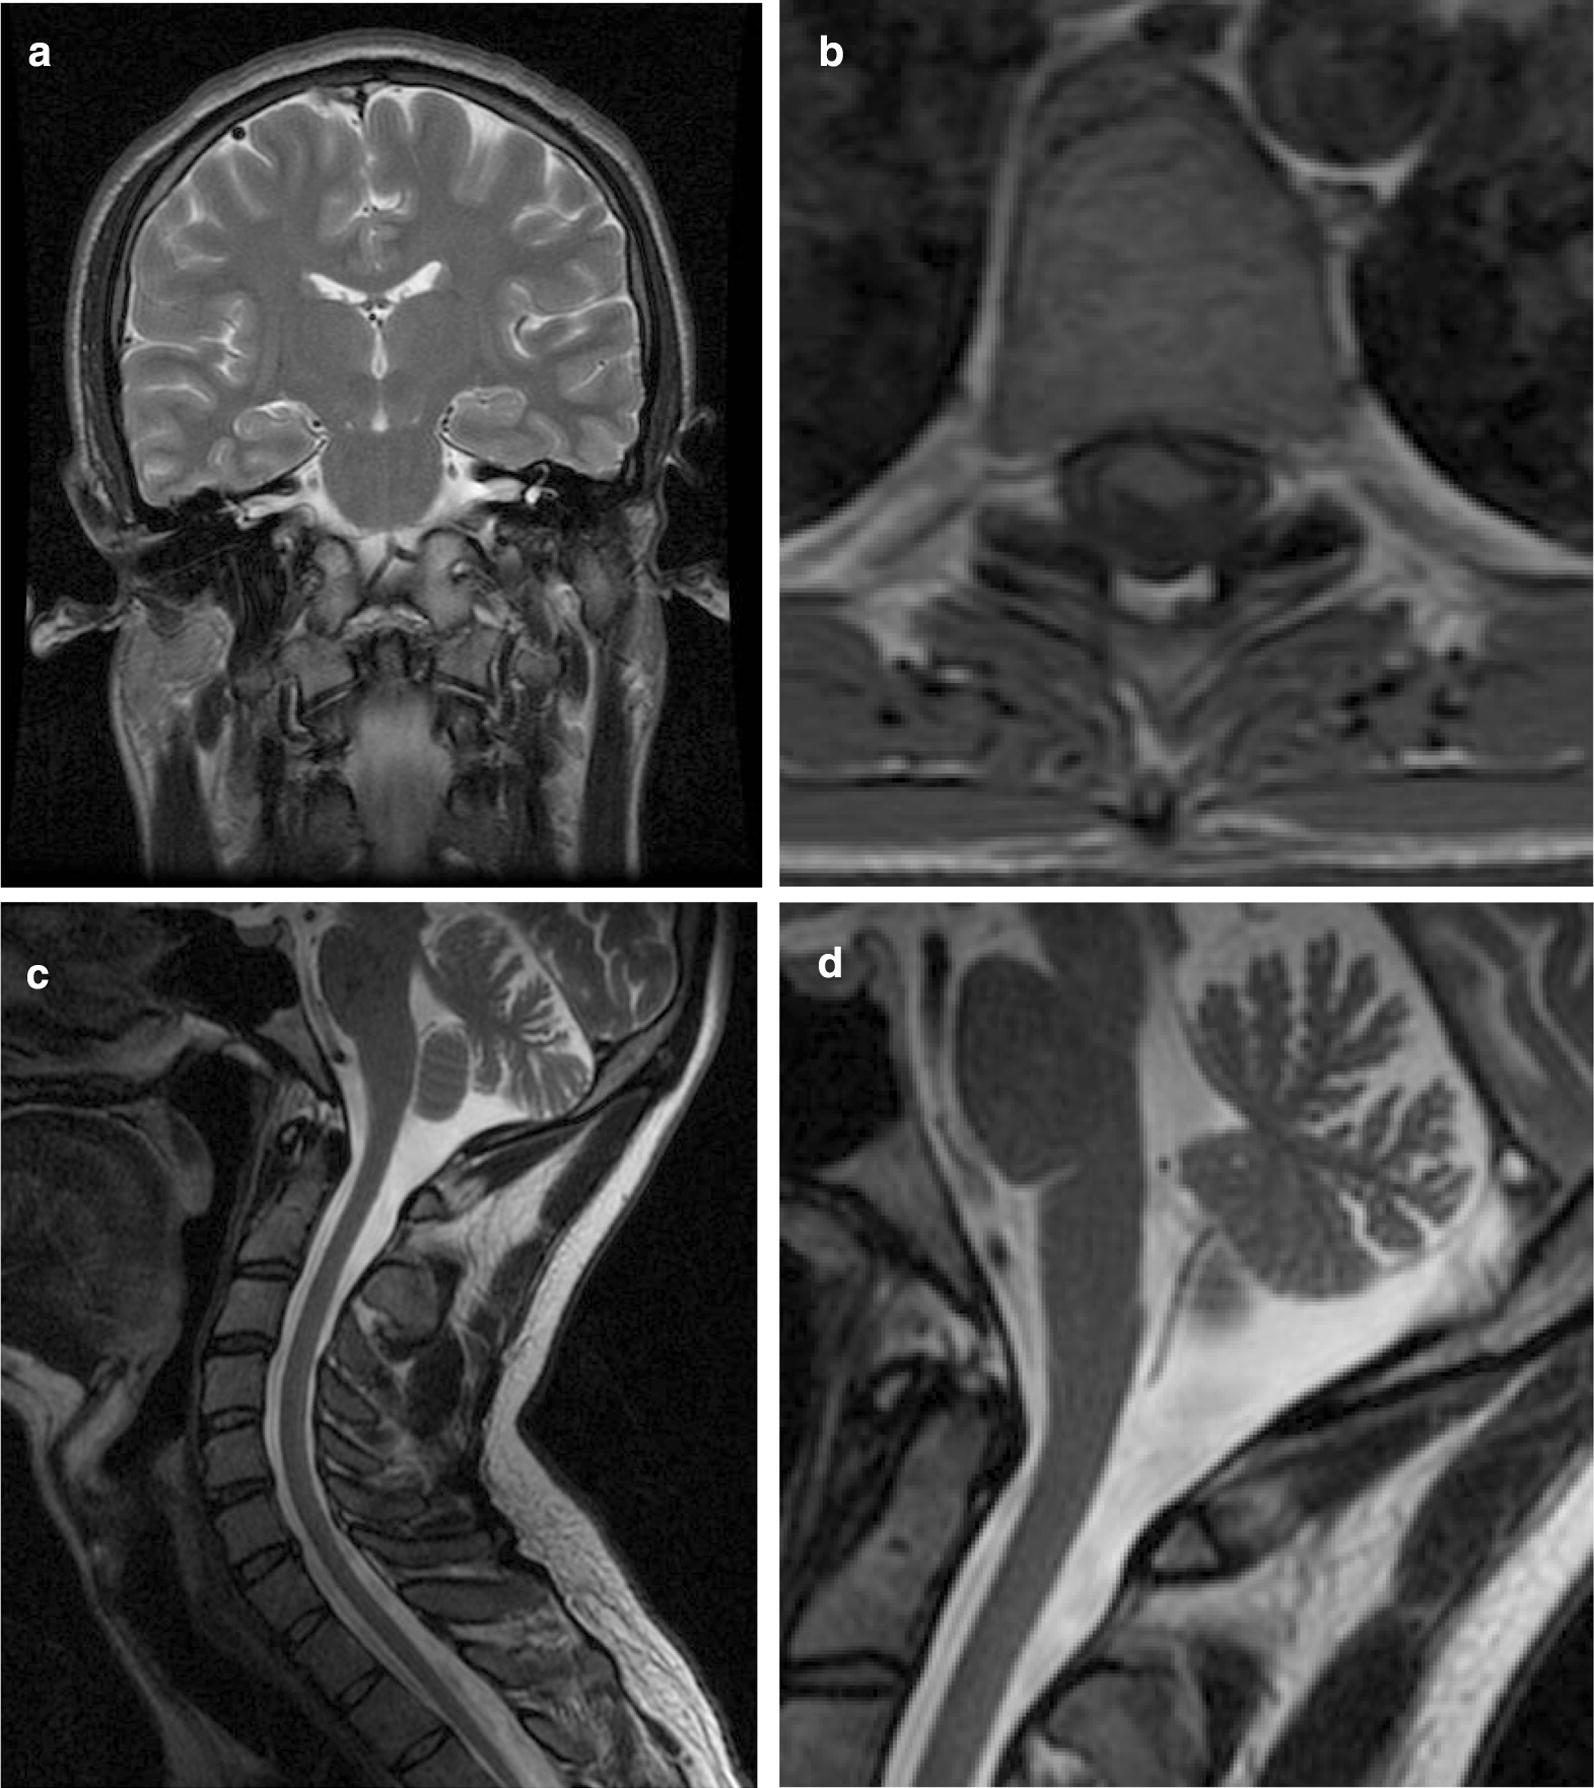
MRI images from subject II:4. a T2-weighted coronal slide showing normal brain parenchyma including brain stem, the inner ears and the vestibulocochlear nerves. b T1-weighted transversal slide of the dorsal spine where flattening of the spinal cord can be seen. c T2-weighted sagittal slide showing cervical kyphosis as well as cerebellar and cervical atrophy. d Detail of the sagittal slide to show the cerebellar atrophy more clearly
